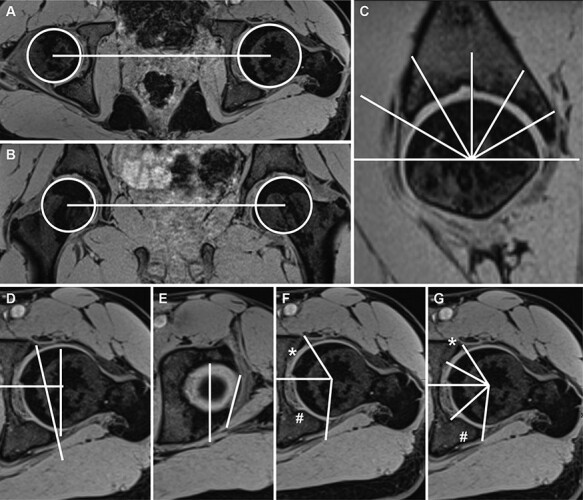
Magnetic resonance imaging (MRI) pelvic alignment and performed measurements: MRI alignment in the (A) axial, (B) coronal and (C) sagittal planes presenting the measured clockwise plains; (D) measurement of the anteversion at the femoral head center, (E) the anteversion at the acetabulum roof, (F) the anterior (*; 3 o’clock) and posterior (#; 9 o’clock) Acetabular Sector Angles (ASA) and (G) the Cartilage Covered Area Angles (CCAA) anteriorly (*; 3 o’clock) and posteriorly (#; 9 o’clock).
